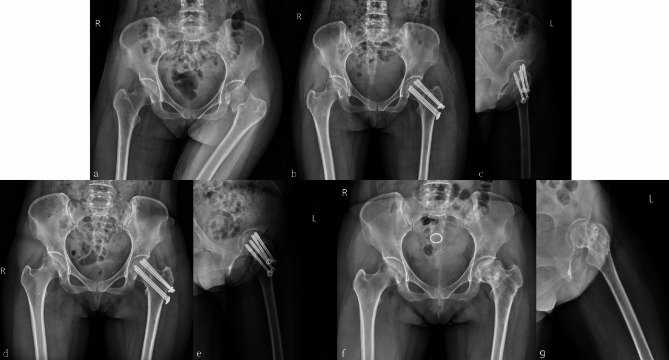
A 37-year-old female patient with left femoral neck fracture was treated with quadruple cannulated screws(QCS). (a) Preoperative anteroposterior X-ray image. (b,c) Postoperative radiographs anteroposterior and lateral images. (d,e) Anteroposterior and lateral X-ray images showing screw withdrawal 1 month after operation. (f,g) Postoperative 15-month anteroposterior and lateral radiographs showing that the screw had been removed owing to discomfort and pain in the patient caused by the irritation of the soft tissue by the screw head on the lateral side
